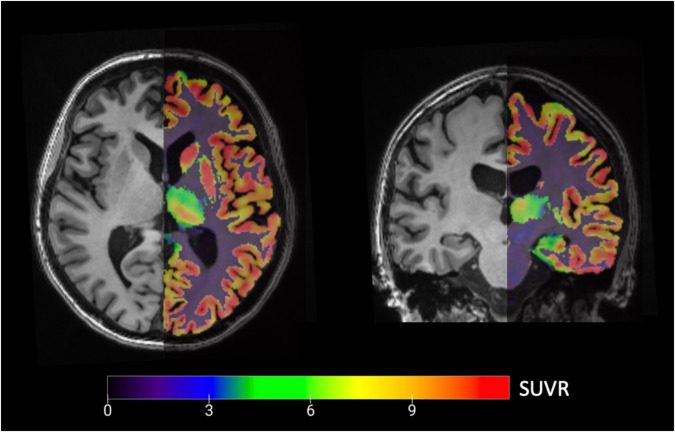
PET-MR imaging. Axial (left, through the prefrontal volume-of-interest) and coronal (right, through the hippocampal volume-of-interest) overlay of a partial volume corrected ^11^C-UCB-J SUVR image (right subparts) registered to the anatomical T1-weighted background for delineation.
